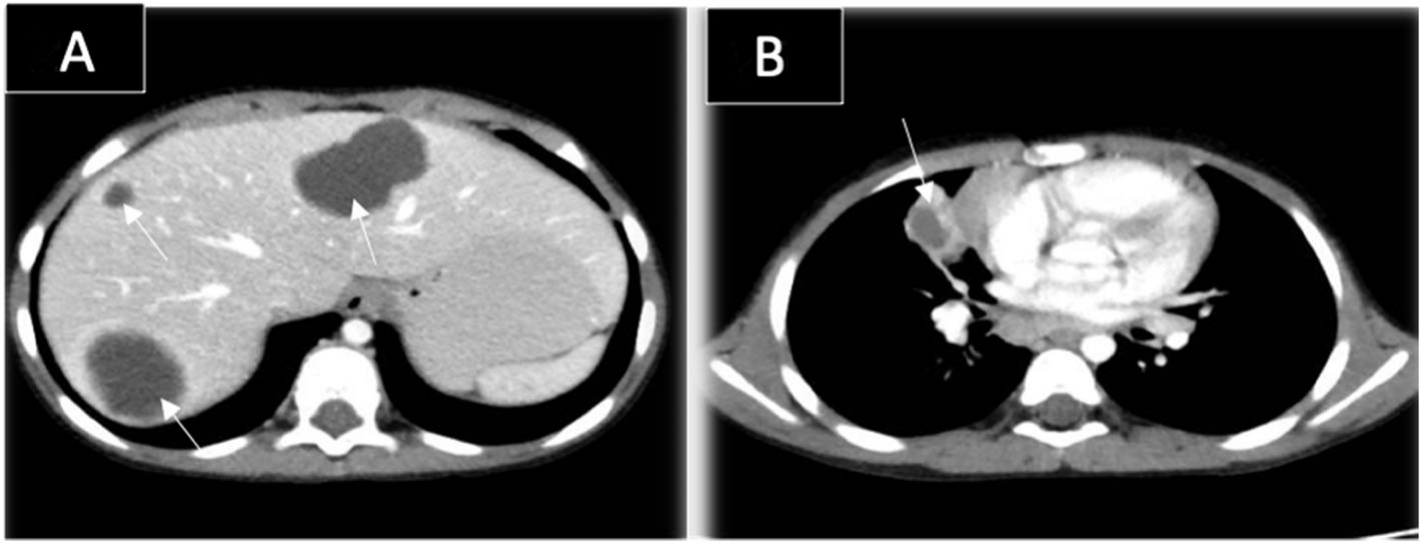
Enhanced axial CT scan of the chest and abdomen. a: showing multiple liver cysts (arrows). b: showing a Multi-loculated cystic lesion in the medial segment of the right middle lung lobe with a surrounding area of collapse consolidation (arrow); representing complicated hydatid cyst
